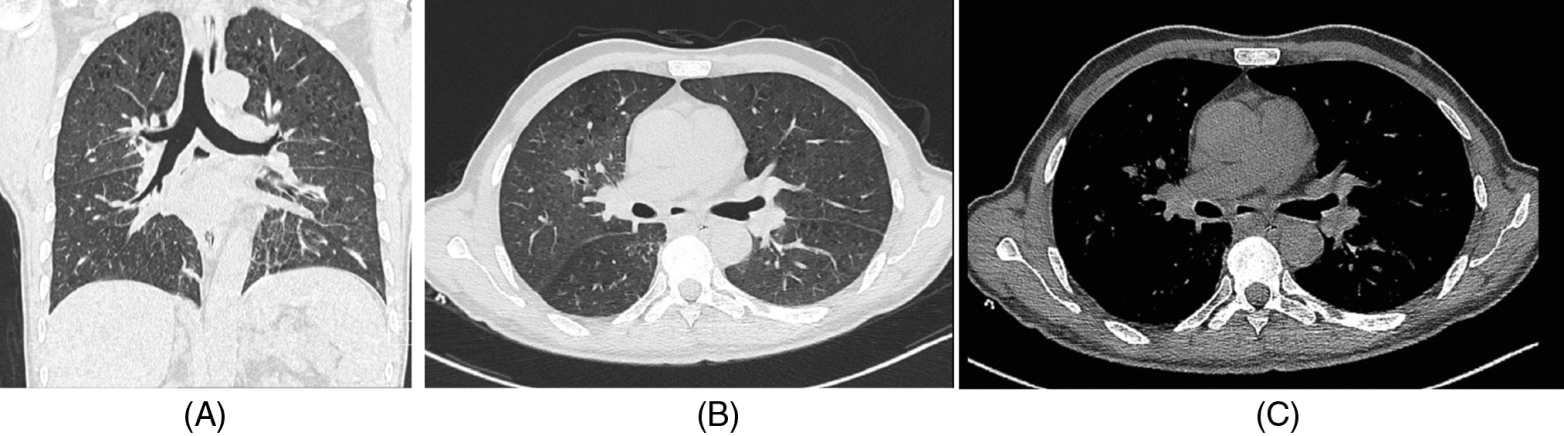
(A) Chest computed tomography longitudinal section, (B) lung window and (C) mediastinal window showed gas collections within the middle mediastinum below the tracheal carina.
